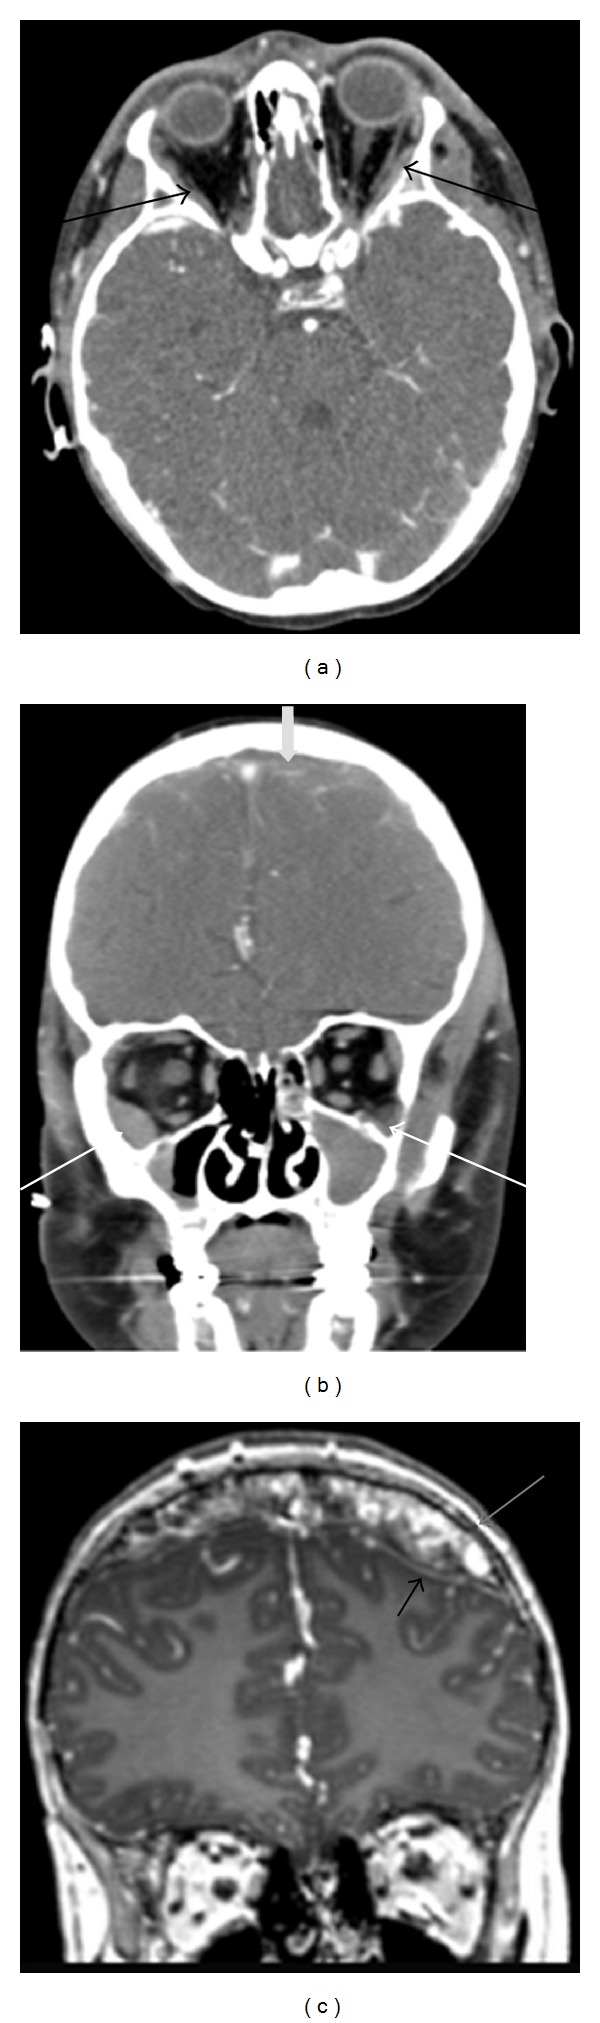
7 years old with history of treated neuroblastoma now with metastatic disease. Axial (a) and coronal (b) postcontrast CT images show ill-defined, slightly hyperattenuating soft-tissue masses along the inferior/lateral periorbital bones (black arrows and thin white arrows) and dural surfaces (thick grey arrow). MR coronal T1-weighted postcontrast image (c) also shows the diffuse, enhancing soft-tissue mass (grey arrow) extending along the dural surfaces (black arrow).
